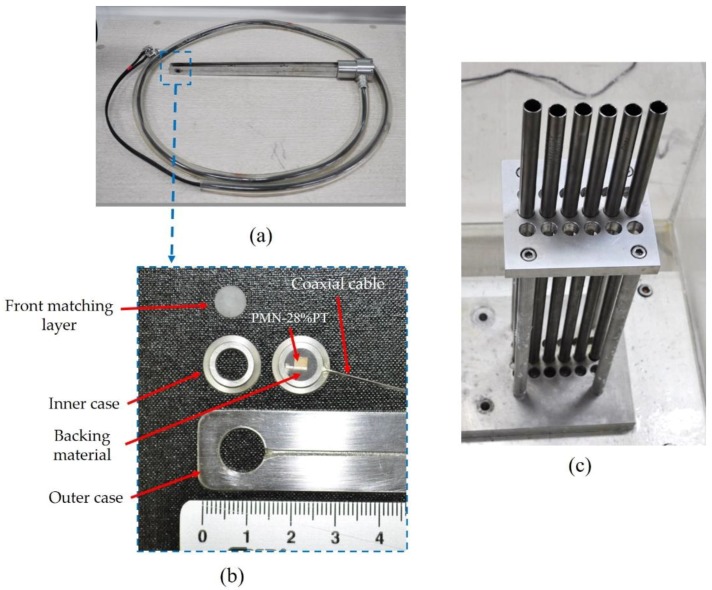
Newly developed PMN-28%PT-based ultrasonic transducer for NDE of PWR fuel rods: (a) Prototype ultrasonic transducer; (b) magnified image of ultrasonic transducer and its components; (c) PWR fuel rod assembly samples.
